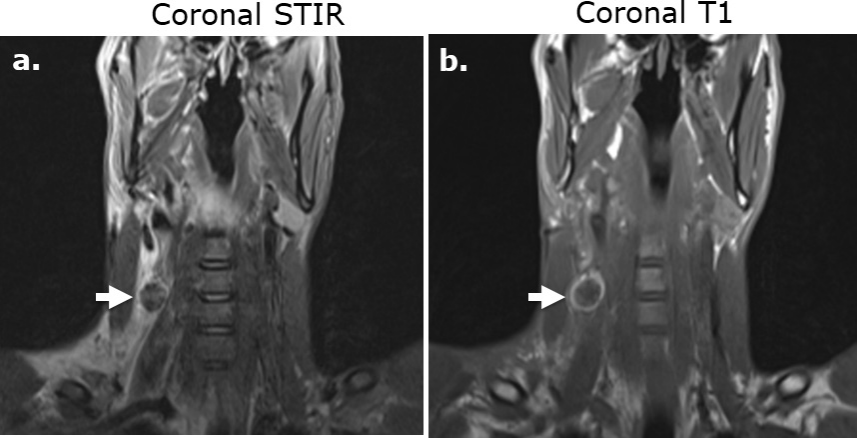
Subcutaneous Surgicel collection following stab wound injury and superficial dissection on MRI. Coronal STIR (a) and coronal T1-weighed (b).) images of the neck in a 29-year-old male following a stab wound injury with Surgicel retained at the surgical site to promote hemostasis. The Surgicel material is nearly uniformly low in *T*
